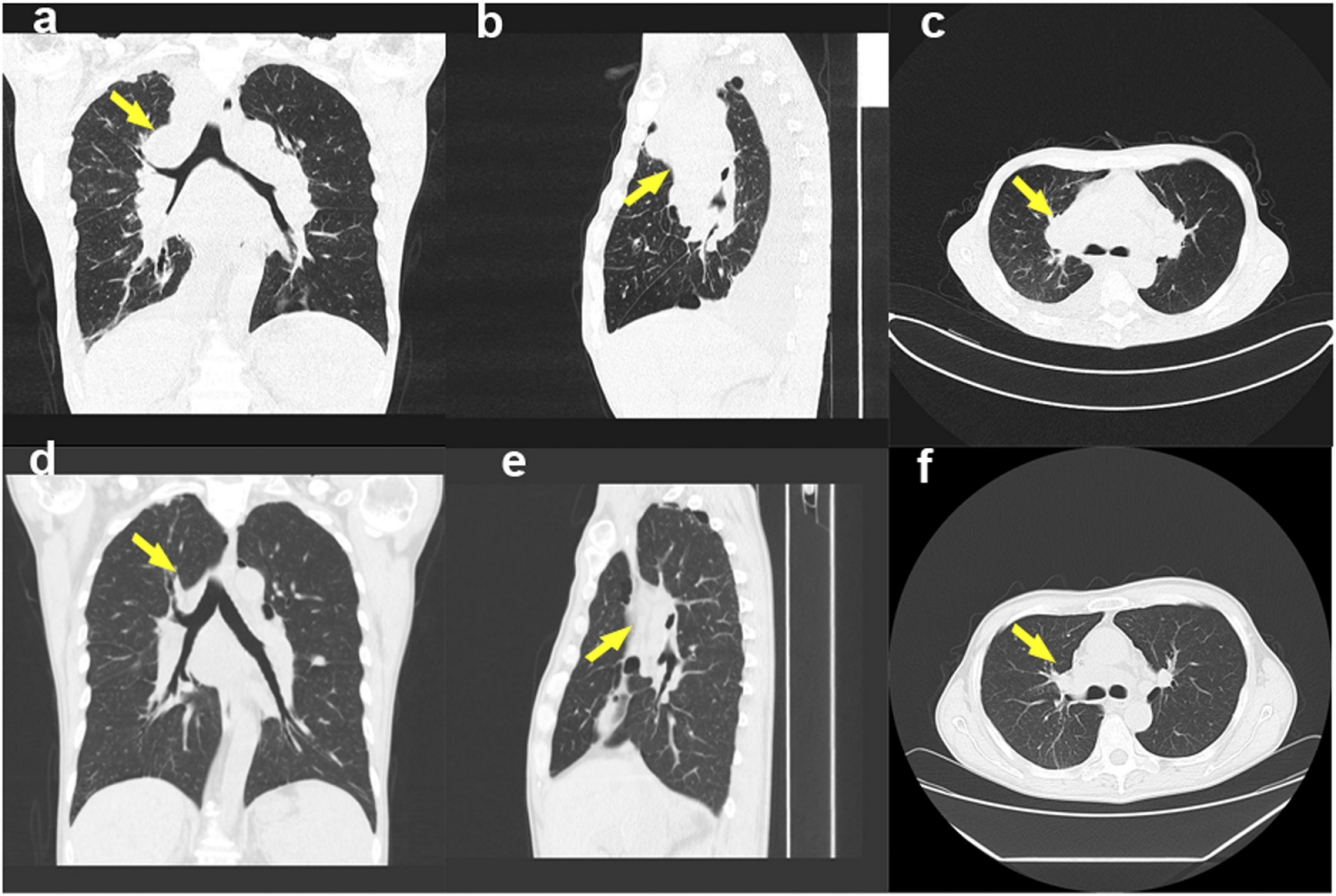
Chest CT before (a)–(c) and after (d)–(f) chemotherapy. (a) and (d) coronal, (b) and (e): sagittal, (c) and (f): axial; yellow arrow, upper right mediastinal mass.
